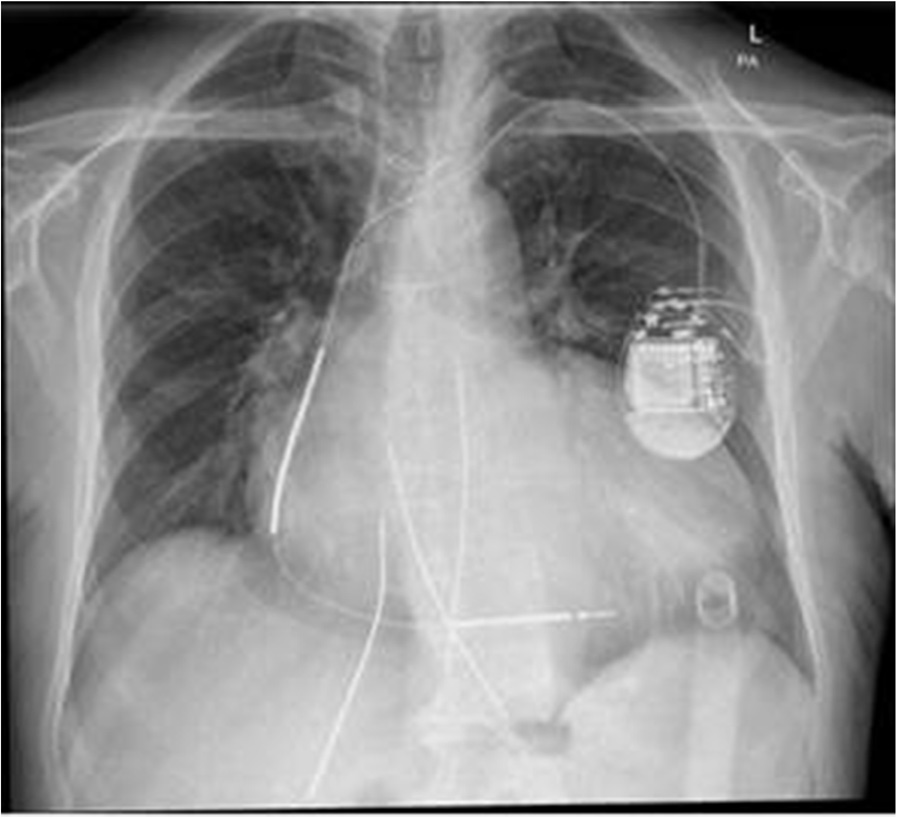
Chest X-ray of the patient on the first postoperative day.
